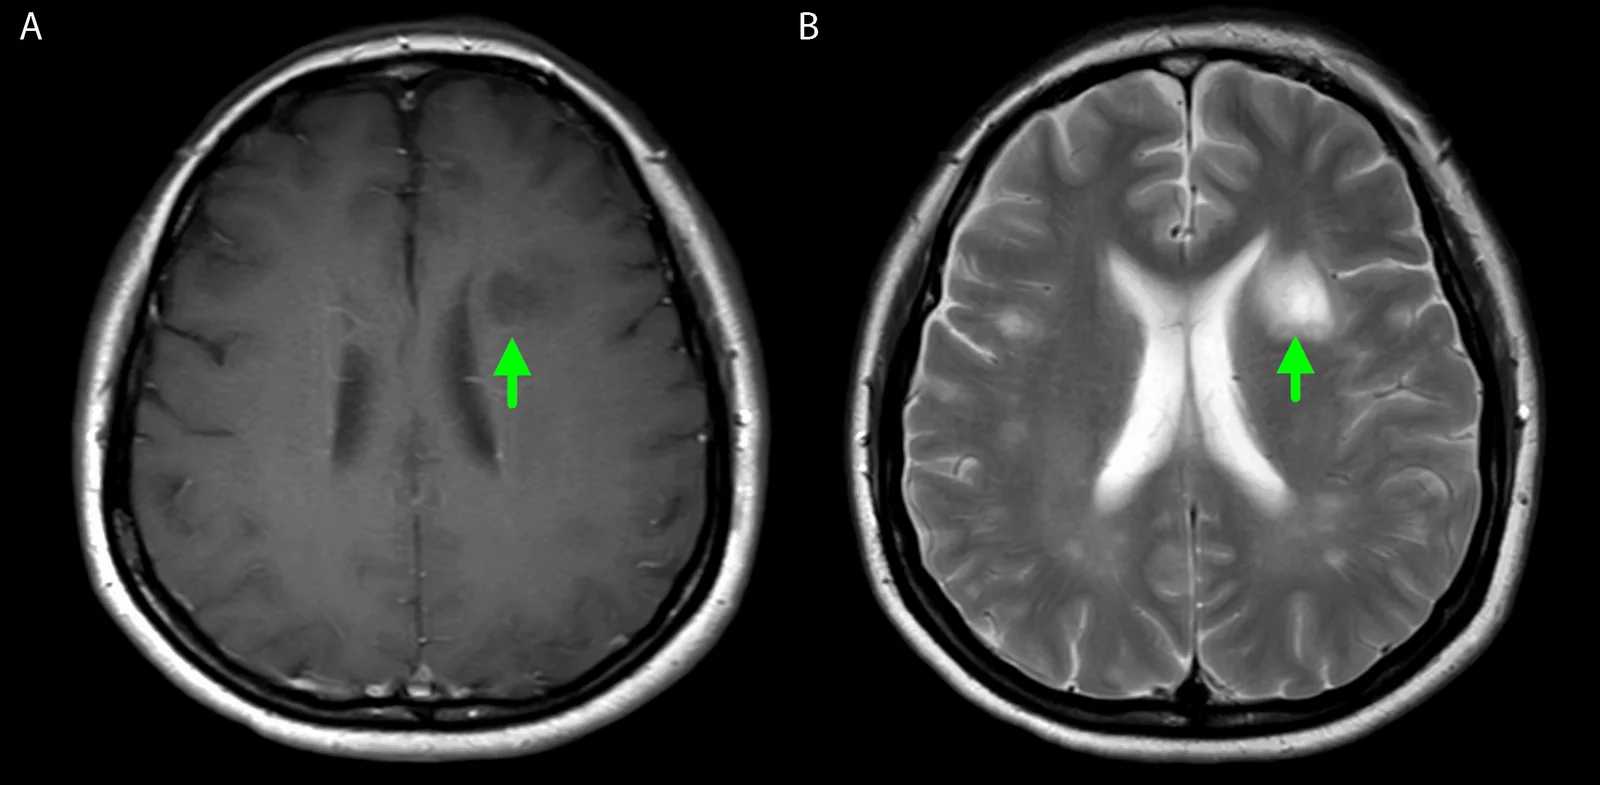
Repeat MRI brain on day 203 after initial COVID-19 vaccination post-contrast T1 (A), non-contrast T2 (B), demonstrating a new non-enhancing T2 hyperintense lesion involving the left corona radiata/centrum semiovale (arrows), suspicious for a new demyelinating lesion, as well as interval decrease in conspicuity of the numerous, previously demonstrated parenchymal lesions, with resolution of associated contrast enhancement. The new lesion suggests a relapsing MOGAD phenotype rather than monophasic ADEM. Repeat MRI brain on day 203 after initial COVID-19 vaccination post-contrast T1 (A), non-contrast T2 (B), demonstrating a new non-enhancing T2 hyperintense lesion involving the left corona radiata/centrum semiovale (arrows), suspicious for a new demyelinating lesion, as well as interval decrease in conspicuity of the numerous, previously demonstrated parenchymal lesions, with resolution of associated contrast enhancement. The new lesion suggests a relapsing MOGAD phenotype rather than monophasic ADEM. ADEM: Acute disseminated encephalomyelitis; MOGAD: myelin oligodendrocyte glycoprotein antibody-associated disease
